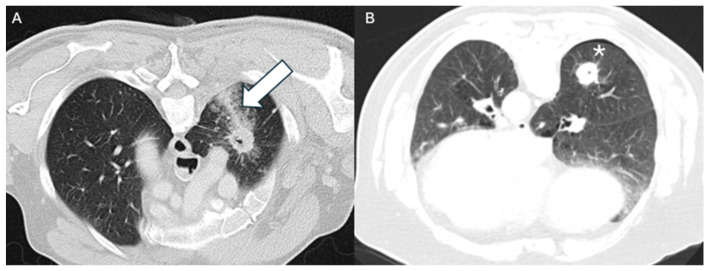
Representative axial CT images demonstrating the inverse relationship between pulmonary hemorrhage and PTX. (A) Axial CT image (lung window, 2 mm slice thickness, 1 mm increment) shows the intrapulmonary lesion with perilesional hemorrhage along the needle trajectory (arrow). No PTX is visible. (B) Corresponding CT image demonstrates the intrapulmonary lesion without adjacent hemorrhage. A narrow PTX is present along the needle path (asterisk).
